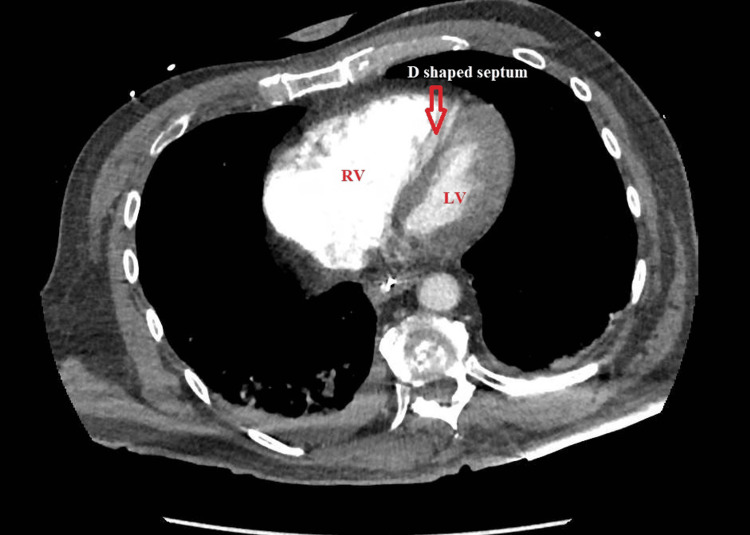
Axial chest computed tomography angiography image demonstrating flattening of the interventricular septum (arrow), resulting in a “D-shaped” left ventricle consistent with acute right ventricular pressure overload.
